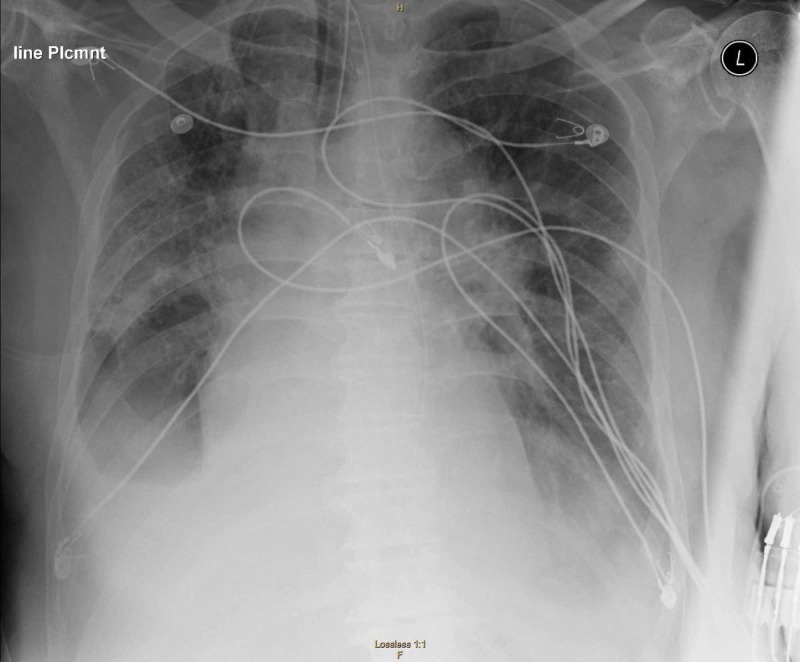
Chest X-ray shows multifocal airspace opacities and “ground-glass opacities”, characteristic signs of COVID-19 infection.
